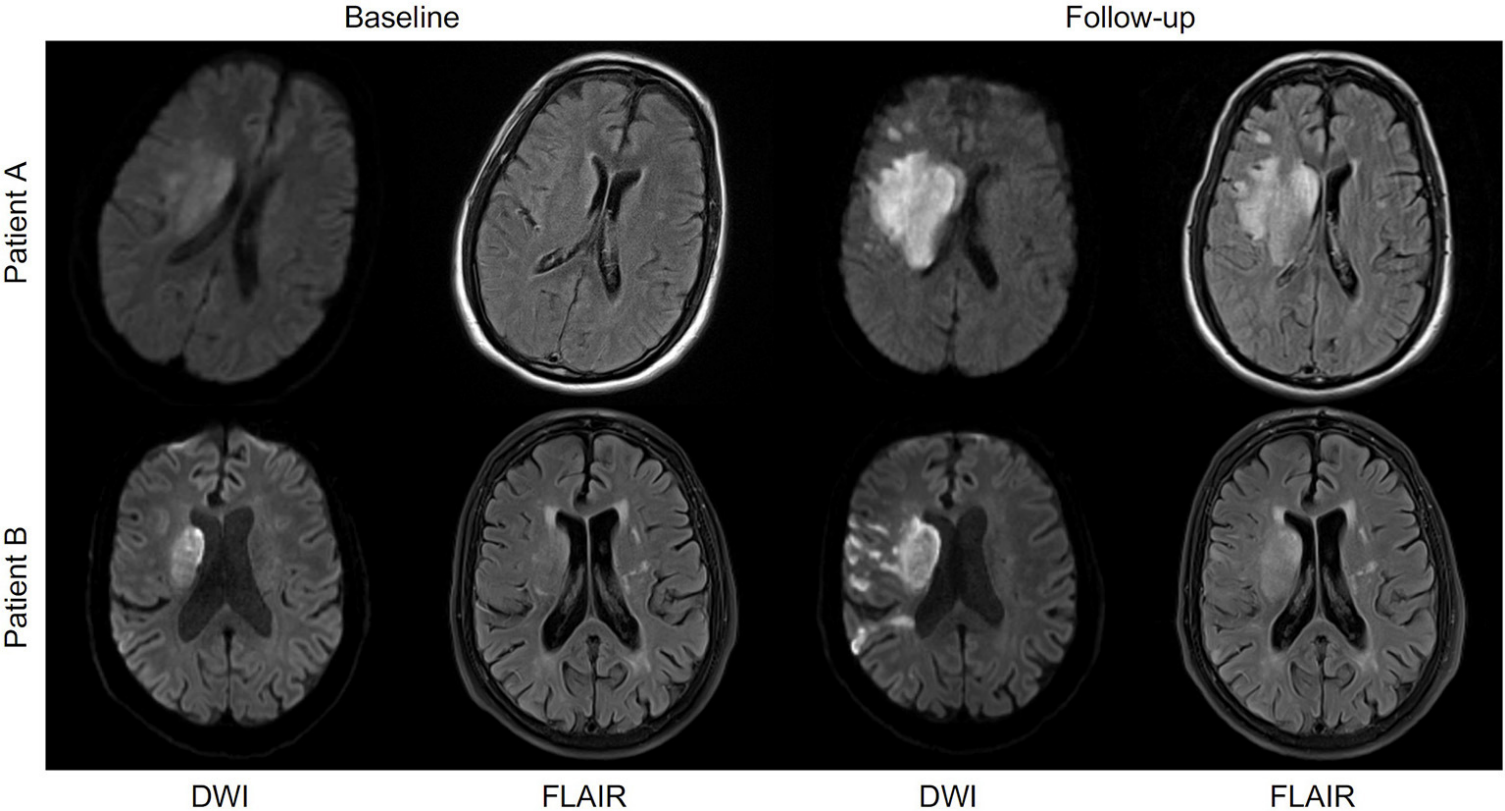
Patients A and B represent cases with comparable stroke evolution yet differing evolution of FLAIR hyperintense vessels (FHVs) between baseline and follow-up imaging. Both 64-year-old males with a right-sided M1 occlusion. Patient A additionally had an occlusion of the ipsilateral internal carotid artery (ICA). The baseline lesions were comparable in terms of volume and pattern (predominantly basal ganglia involvement and lesion size up to 20 ml). Their baseline National Institutes of Health Stroke Scale (NIHSS) score was also comparable (15 for patient A and 14 for patient B). The baseline extent of FHVs was different between the patients (30 in patient A and 50 in patient B). Both patients received placebo, and neither of them experienced a recanalization by the time of follow-up. There was a pronounced difference in the dynamics of FHVs between the patients, with patient A showing no more visible FHVs at follow-up, whereas the extent of FHVs in patient B actually increased from 50 to 55. Their outcome, however, was the same (both had a 90-day mRS of 4), and their final lesion volumes were almost identical (patient A 52 ml and patient B 59 ml).
